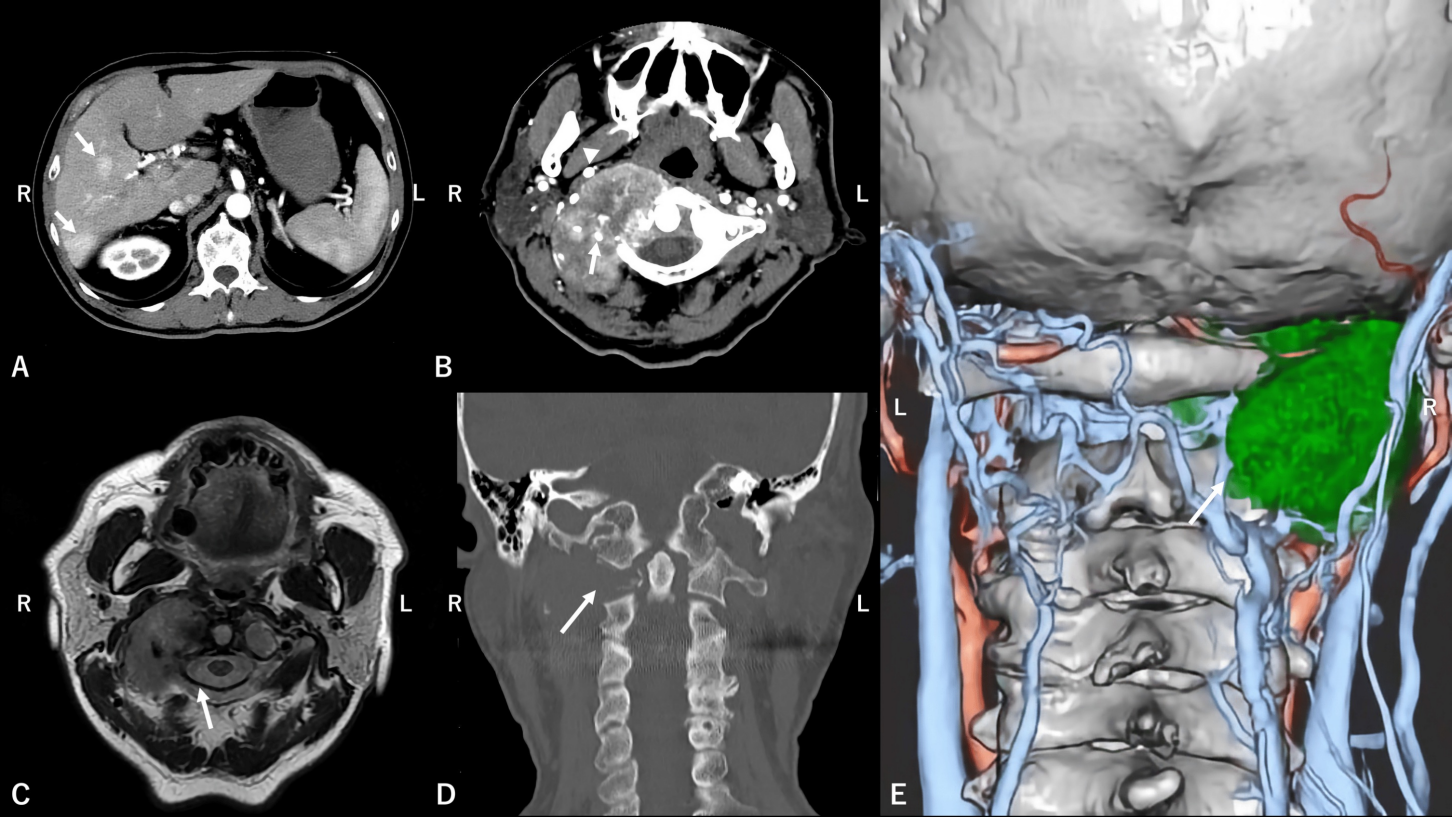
Preoperative imaging findings (A) Dynamic contrast-enhanced abdominal CT: Multiple liver masses (arrow) exhibiting arterial-phase enhancement and lack of enhancement in the portal venous phase, leading to a diagnosis of primary hepatocellular carcinoma (HCC). (B) Contrast-enhanced cervical CT: A 5.5 cm lytic tumor is identified in the right C1 lateral mass, extending to the anterior and posterior arches and involving the paravertebral muscles. The right vertebral artery (arrow) is completely involved, and the internal carotid artery (arrowhead) is anteriorly compressed by the tumor. (C) Plain cervical MRI T2 weighted image: An extradurally extending tumor of the right C1 lateral mass is observed, but there is no compression or deformation of the spinal cord (arrow). (D) Plain cervical CT showing complete destruction of the right C1 lateral mass (arrow), suggesting severe instability. (E) Reconstructed enhanced CT: The tumor extends to the posterior aspect of the C2 lamina (arrow), rendering pedicle screw placement unfeasible.
